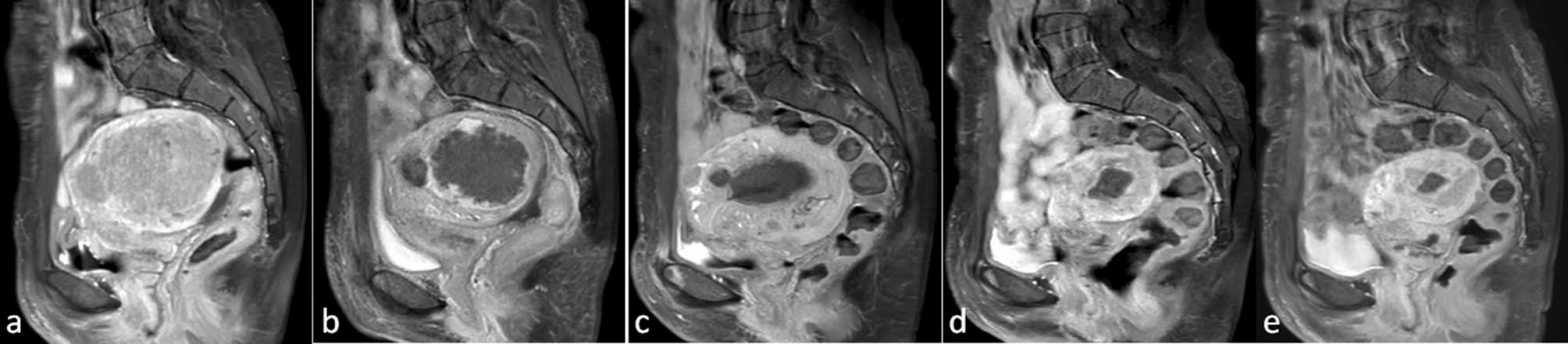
A 45-year old patient with symptomatic uterine fibroids presented with menstrual complaints (hyper-dysmenorrhea), urination urge, pain/pressure in the pelvic region and was treated by USgHIFU in our hospital. Three weeks after HIFU, considerable symptom relief was observed. Representative MRI-images (a–e, contrast-enhanced T1-weighted in sagittal plane) of the largest uterine fibroid are shown over time before and after HIFU treatment: (a) baseline with initial fibroid size of 6.2 × 5.4 cm; (b) one day post-HIFU large treated region showed no contrast enhancement indicative of effective ablation*;* (c–e) A continuous reduction in fibroid volume after HIFU-treatment was observed, this was 66.4%, 84.6% and 90.1% after 6 months, 1 and 2 years, respectively.
